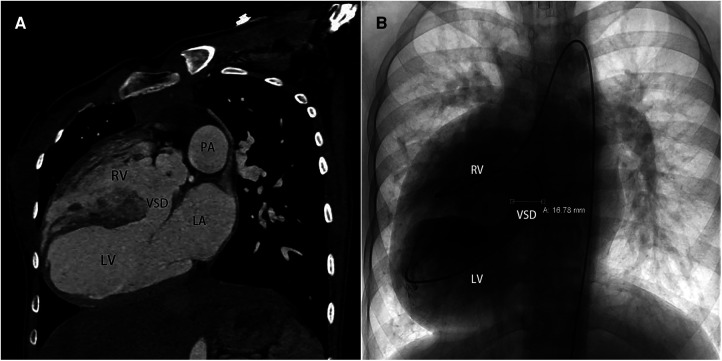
(A) computed tomography angiography pre-operatively in sagittal view, (B) cardiac catheterization in anteroposterior projection. VSD, ventricle septal defect.
